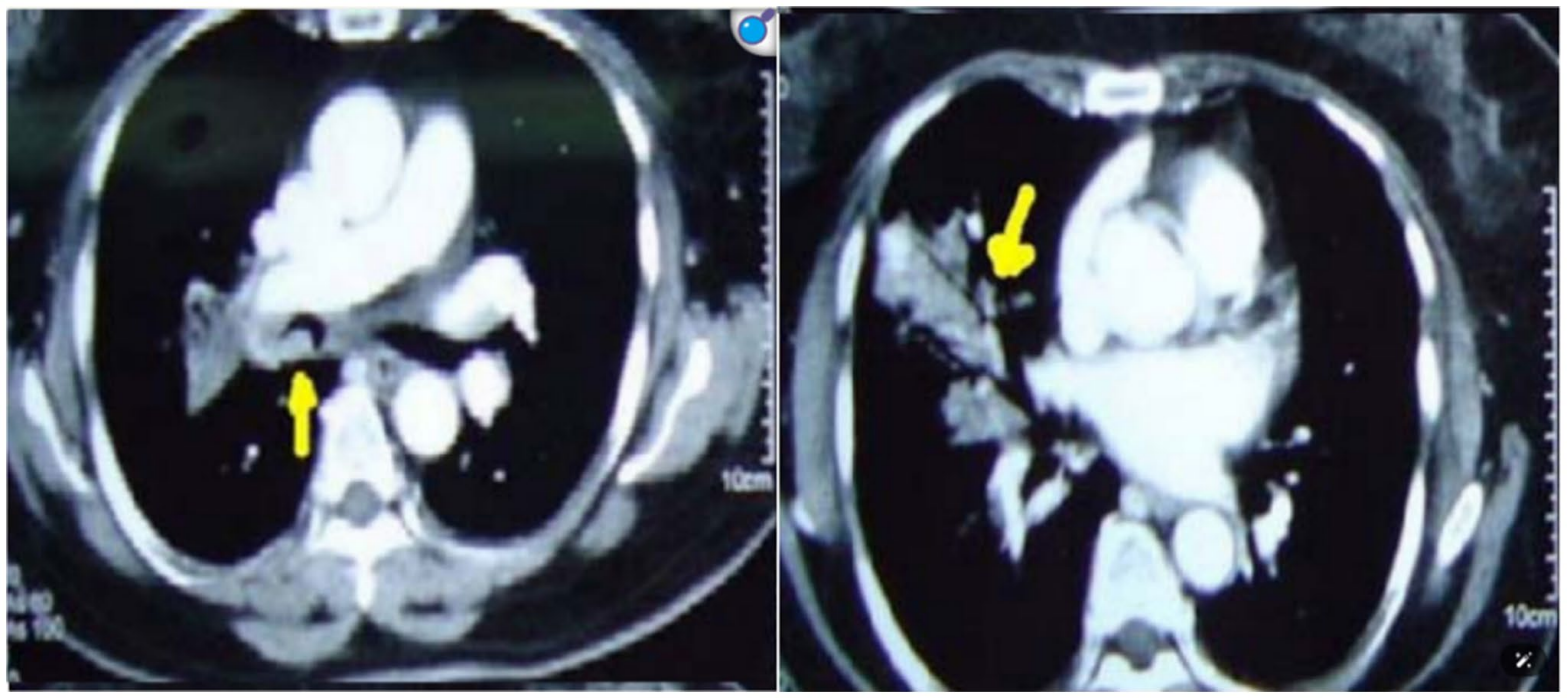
Chest CT image of a patient with tracheobronchial tuberculosis.
